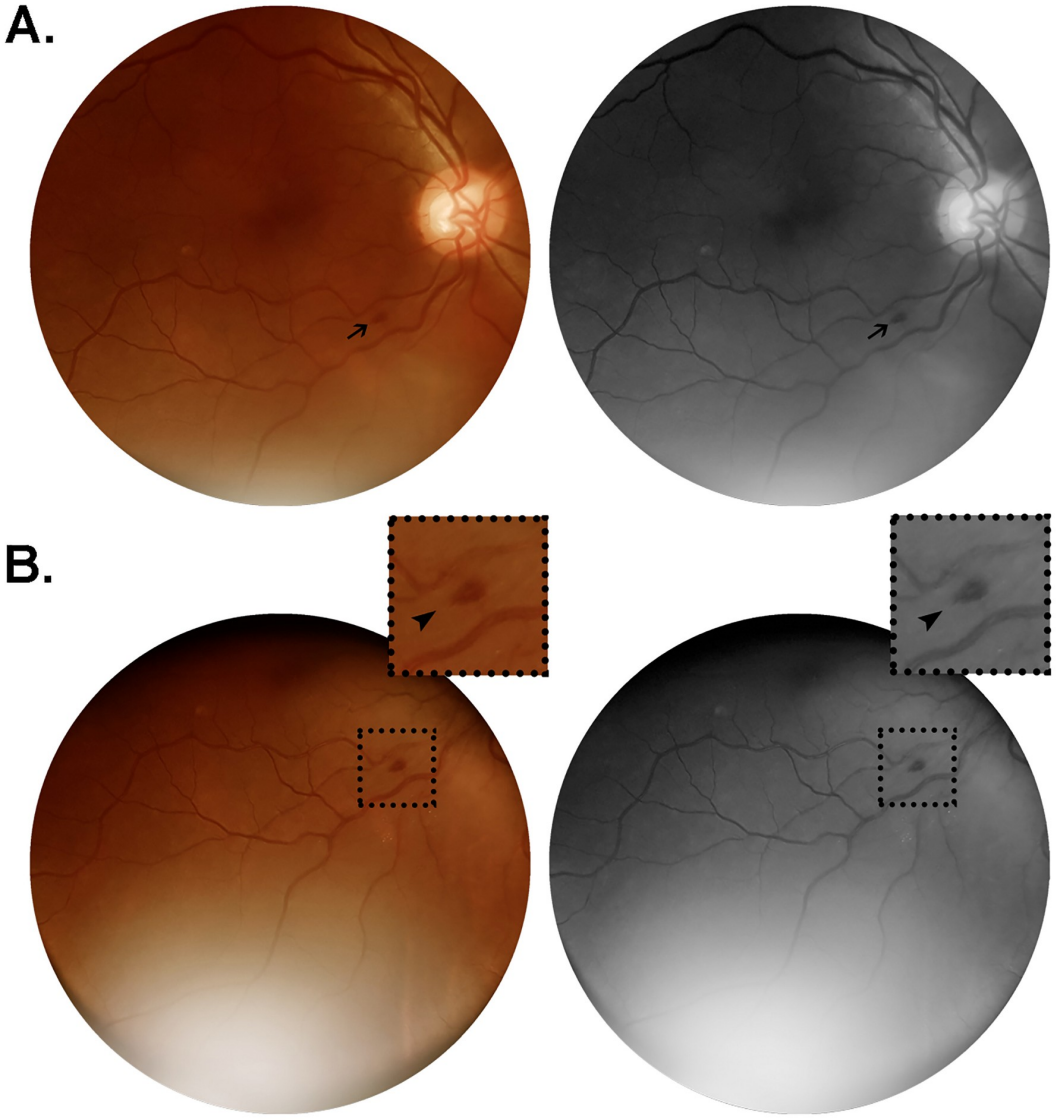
Color and red-free retinal photographs from patient #18 (W.S.). Images acquired on day 16 from symptoms onset, from a 56 year-old man with no comorbidities, admitted on day 13 after onset of a clinical scenario characterized by fever, cough, headache, myalgia and dyspnea. The patient evolved to severe illness, not requiring ICU monitorization nor developing any organ failure. Lung CT at admission revealed bilateral ground-glass opacities predominantly in the periphery, extending to approximately 50% of lung parenchyma. (A) Flame-shaped hemorrhage (arrow) approximately one disc diameter from the optic nerve head, close to the inferior temporal vascular arcade. (B) Same lesion observed from an alternative point of gaze fixation, magnified in the insets (2x; arrowheads).
